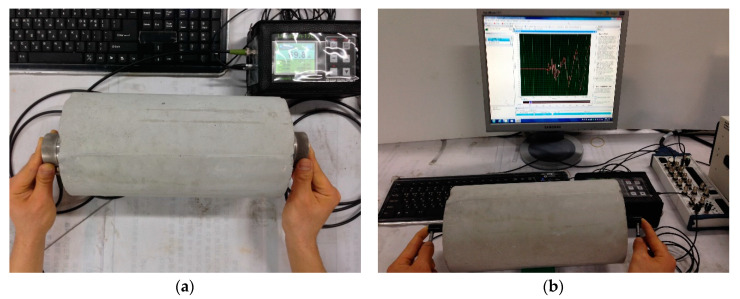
Methods for measuring (a) ultrasonic pulse velocity using a P-wave transducer (MK 954) and (b) ultrasonic shear-wave velocity using an S-wave transducer (ACS T1802).
